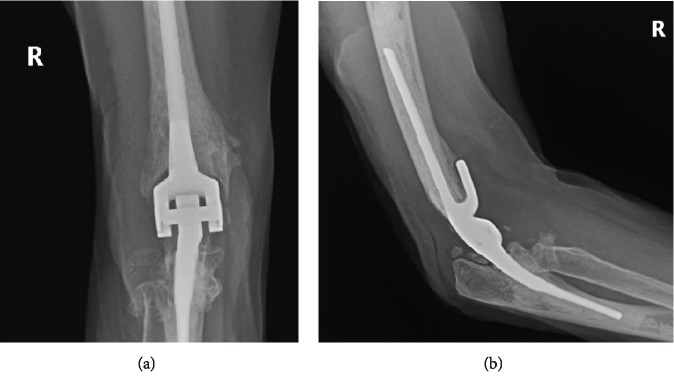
Follow-up AP (a), and lateral (b) X-rays 10 months after HO excision and radiation treatment.
